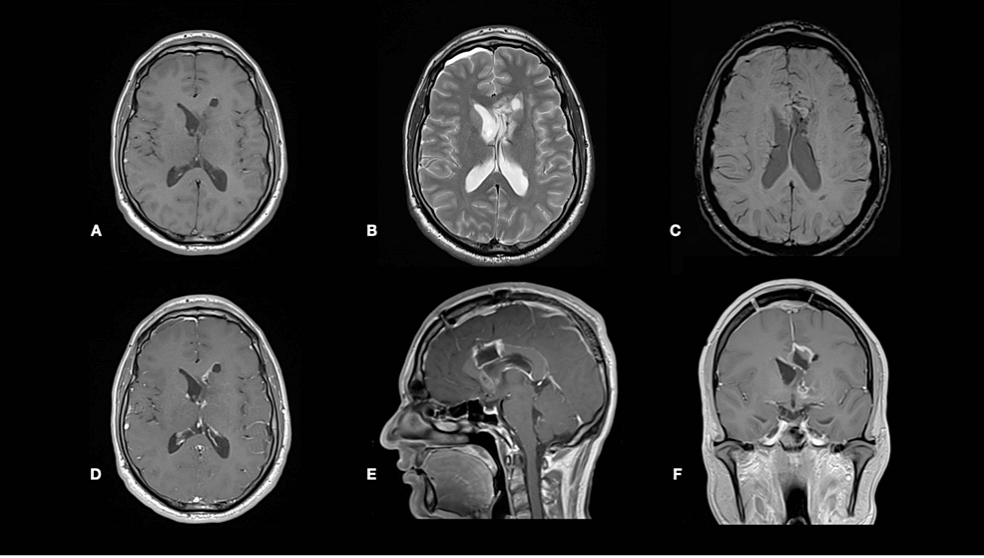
Postoperative brain MRI scan (A-F) after right frontal craniotomy and transcallosal microsurgical resection of the tumor revealed complete resection and resolution of surrounding hemorrhagic changes.
